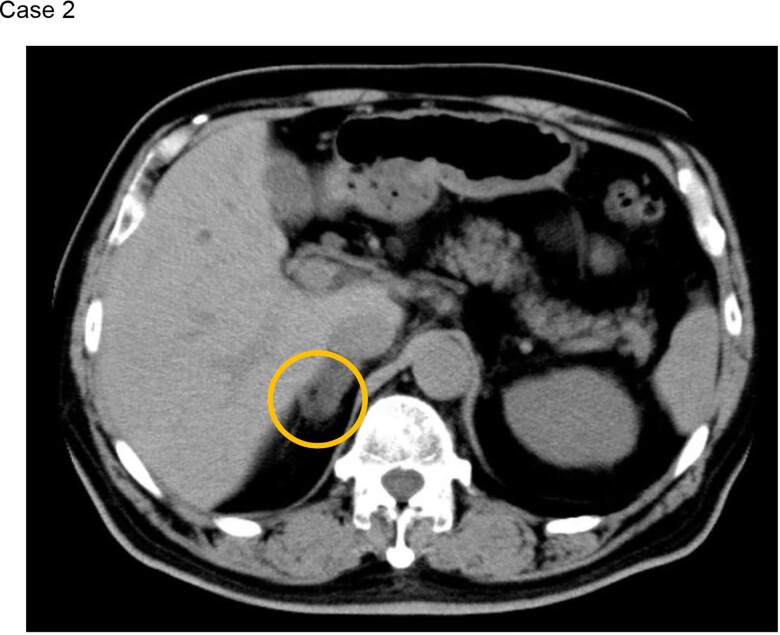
Imaging findings at the time of recurrence (case 2). The image shows computed tomography at age 65. The orange circle indicates a tumor with a diameter of 30 mm in the right adrenal gland.
